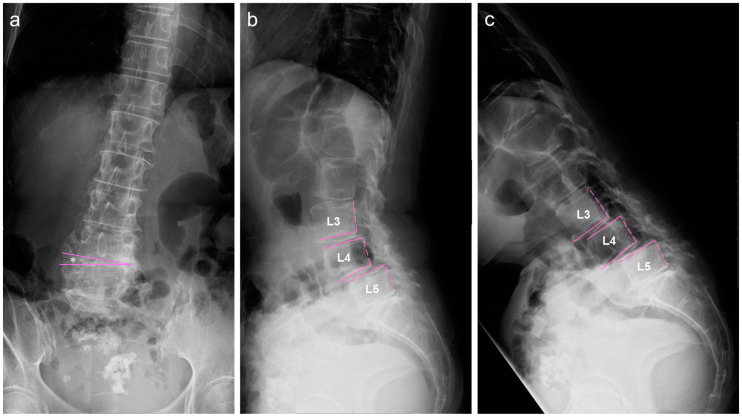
Standing lumbar radiographs at baseline. (a) Antero-posterior view, (b) lateral neutral position, (c) lateral forward-bending position. Straight lines indicate the margin of vertebral endplates, and spotty lines indicate the margin of posterior wall of vertebrae. * indicates 12° of L4 coronal tilt.
